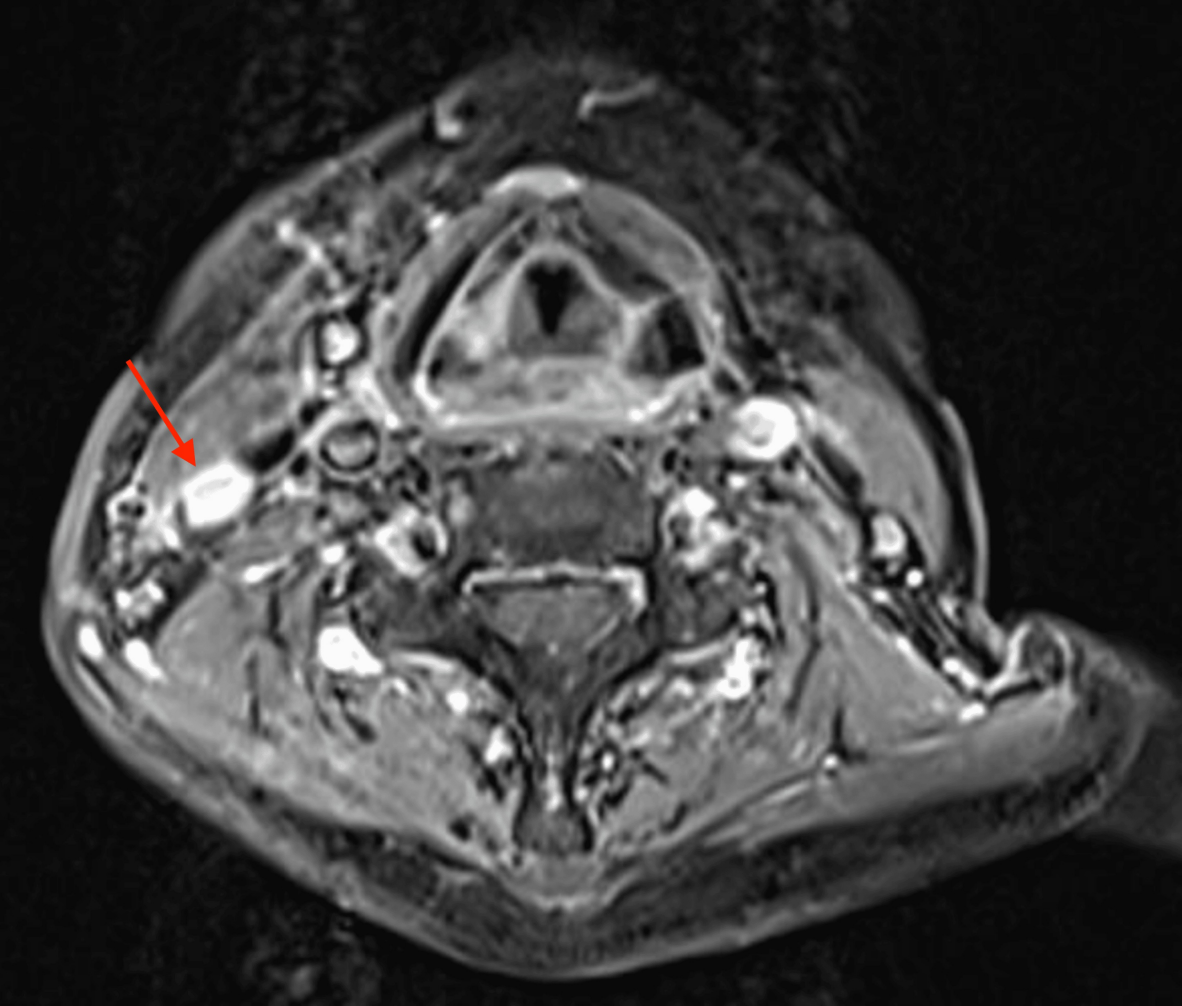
MRI of the neck depicting lymph node metastases to the right at level III, corresponding to an increased FDG uptake on PET-CT as in Figure 6 MRI, magnetic resonance imaging; FDG, fluorodeoxyglucose; CT, computed tomography
